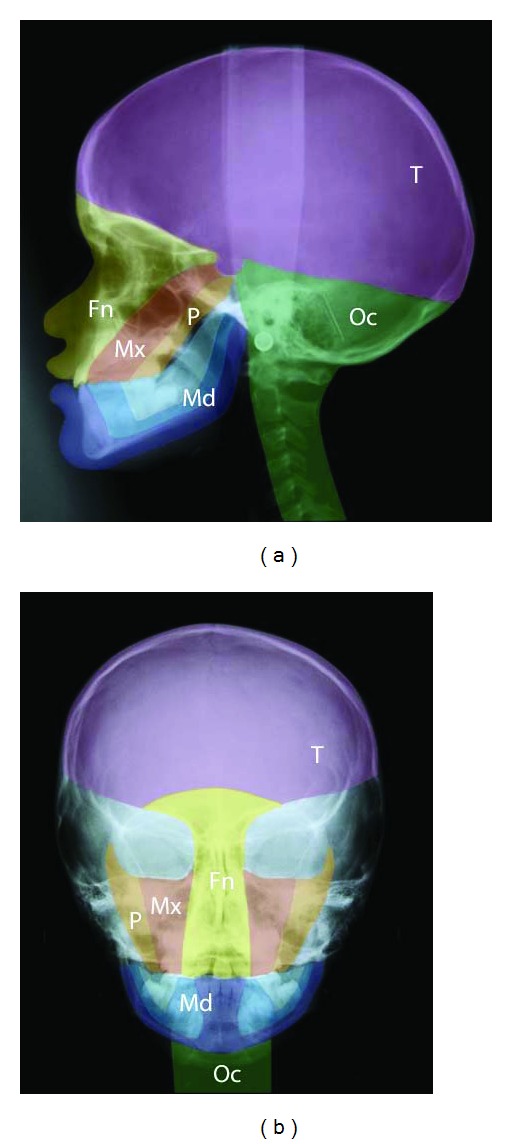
Profile (a) and frontal (b) radiograph of a girl aged 9 years with a single median maxillary central incisor. Different developmental fields are marked on the radiographs. Green: occipital and cervical spine field (Oc). Purple: theka field (T). Light and dark blue: the complete mandibular field (Md). Yellow: frontonasal field (Fn). Red: maxillary field (Mx). Orange: palatal field (P). Note that the sella turcica is a borderline region between fields. This figure is reprinted with permission from European Journal of Orthodontics 2010:32:140-147 [5].
